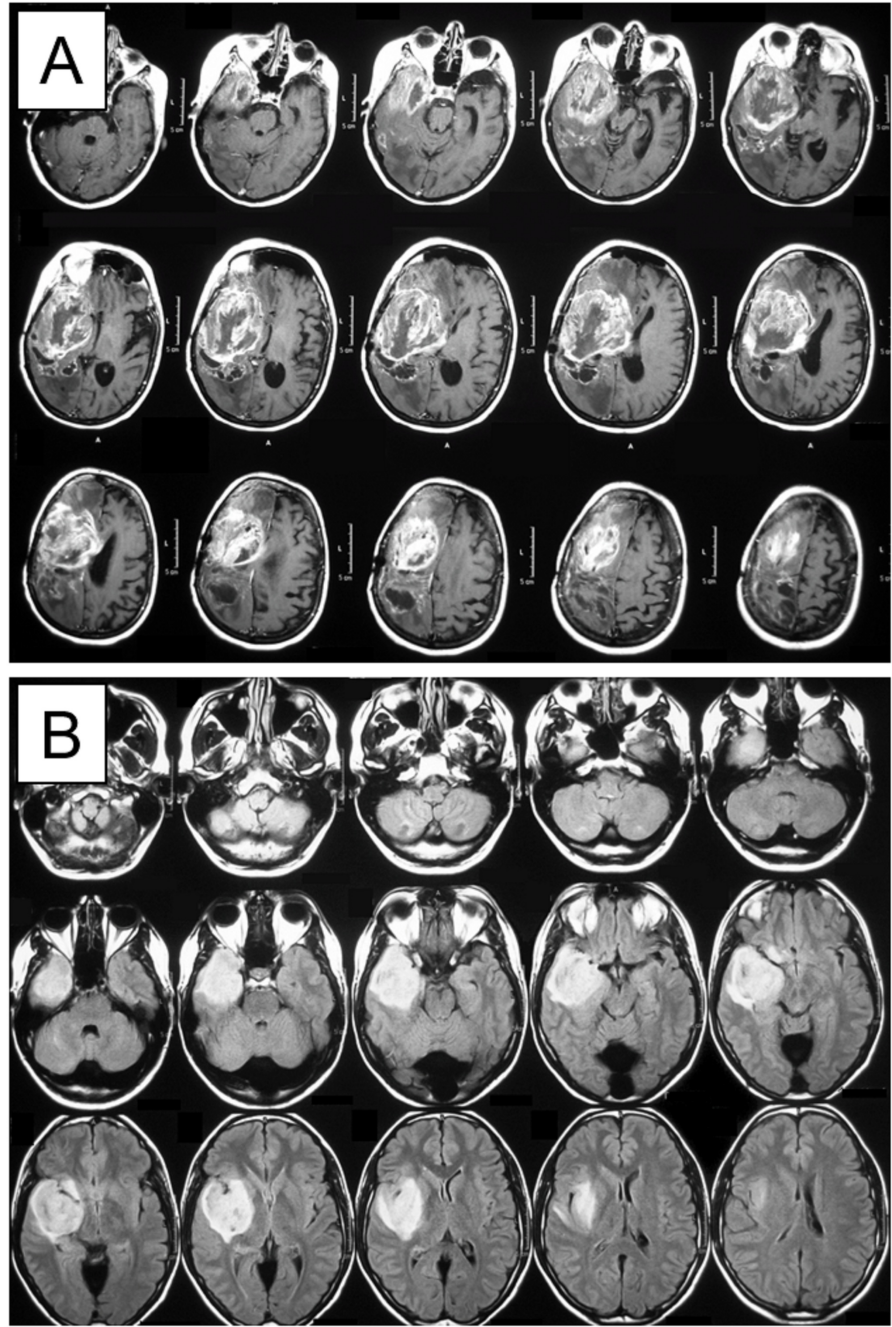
Examples of two MIGs used in the study Magnetic resonance imaging (MRI) of (A) contrast-enhancing tumor on T1-weighted sequence and (B) hyperintense tumor on T2/ Fluid Attenuated Inversion Recovery (FLAIR) sequence Multilobar insular-involving gliomas: MIGs
